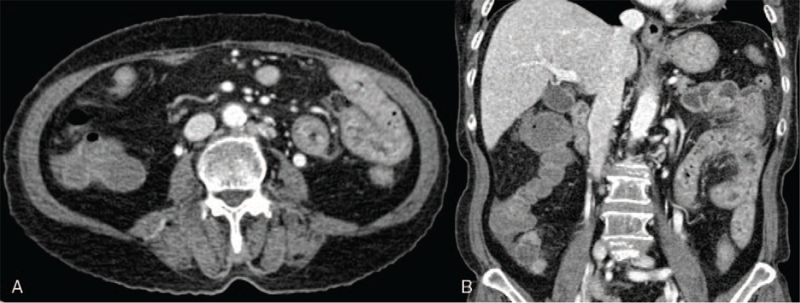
Abdominal computed tomography at presentation reveals edematous wall thickening of the small bowel and loose stools in the colon.
